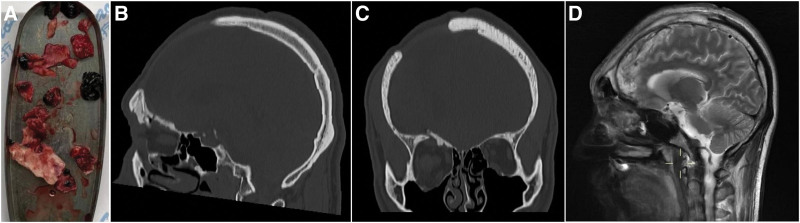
(A) Intraoperative removal of the bone fragments, hematoma, and tissue. (B) Postoperative sagittal reconstructed CT image showing the removal of bone fragments and hematoma. (C) Postoperative coronal reconstructed CT image demonstrating the reconstruction of the anterior skull base and supraorbital wall. (D) MRI sagittal image at postoperative 2 weeks revealing good recovery of the operated area. CT = computed tomography, MRI = magnetic resonance imaging.
